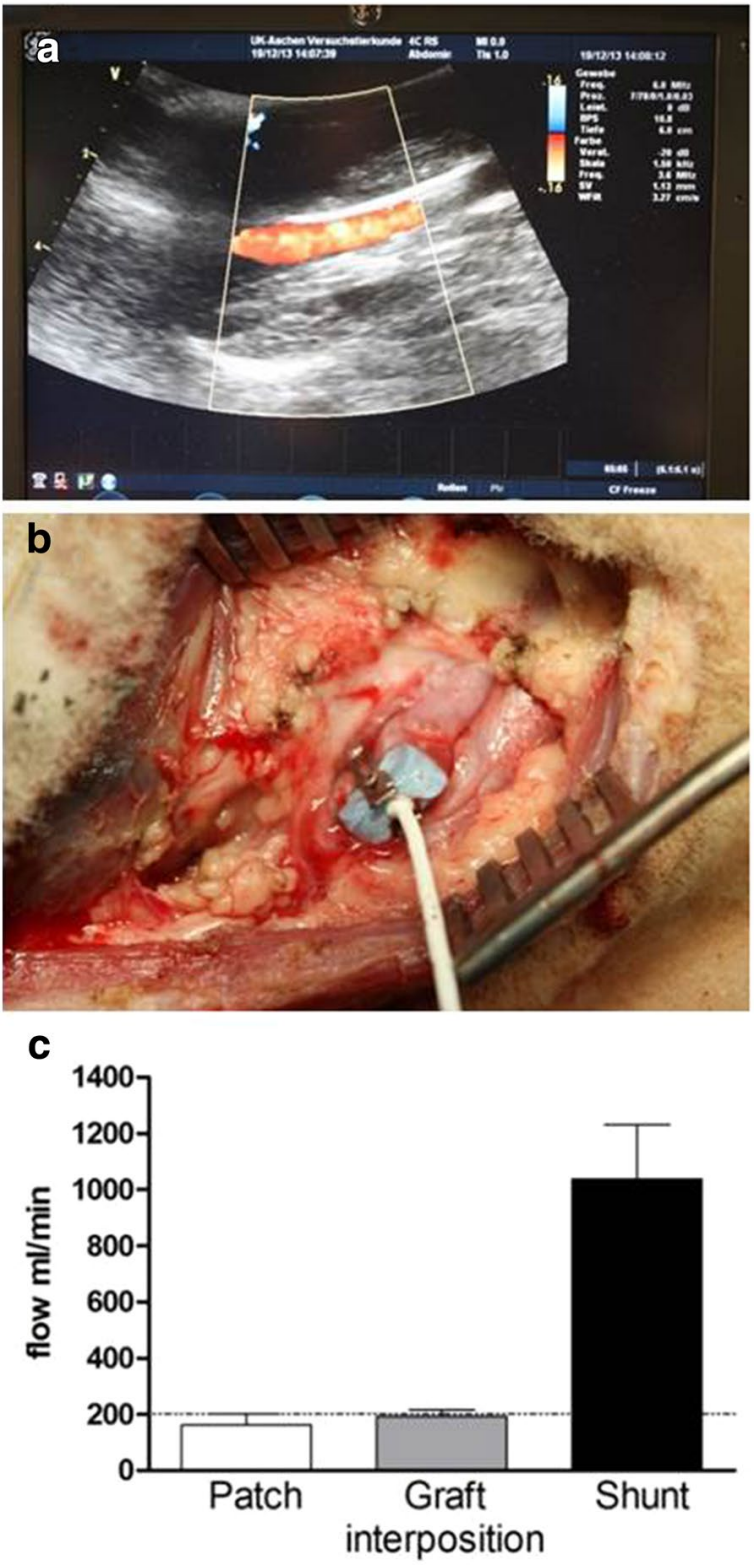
a Color-coded duplex ultrasonography of the common carotid artery. b Blood flow measurement in the carotid artery. The perivascular ultrasonic volume flow-sensor is placed around the carotid artery. c Blood flow at the time point of euthanasia in the common carotid artery after patch angioplasty, graft interposition and straight shunt between the common carotid artery and the external jugular vein. Dotted line represents physiologic blood flow as described in literature
